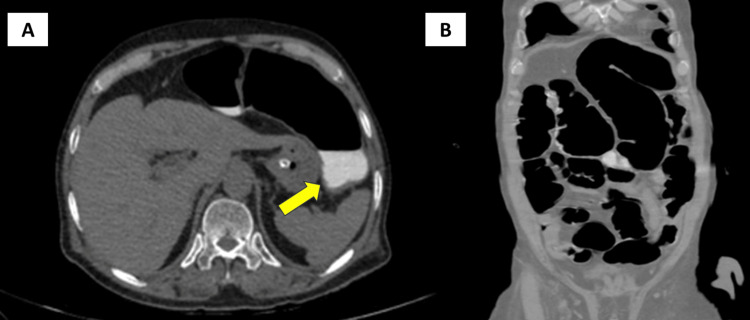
Computerized tomography (CT) of the abdomen and pelvis with contrast (A) The transverse section demonstrates a dilated transverse colon measuring 5.3 cm in its largest diameter with retained positive oral contrast, indicated by a yellow arrow; (B) the coronal section demonstrates dilated large bowel loops with no evidence of a cutoff point and no apparent mechanical obstruction.
